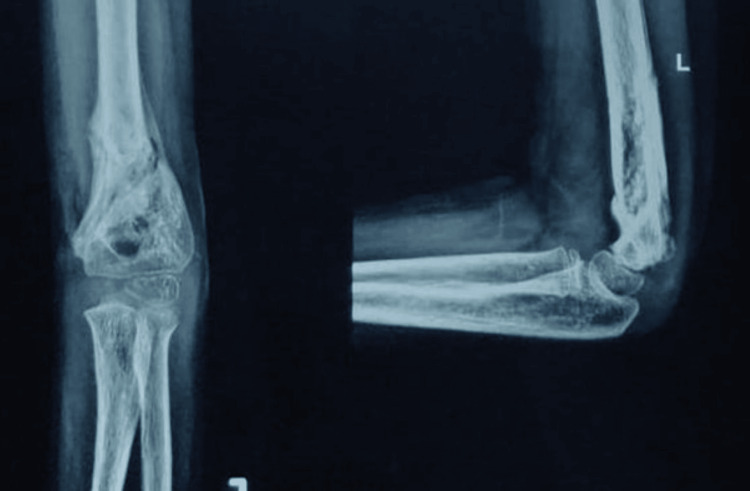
Radiograph of left elbow. Anteroposterior and lateral views after six weeks - implant removal, showing union of fracture.
